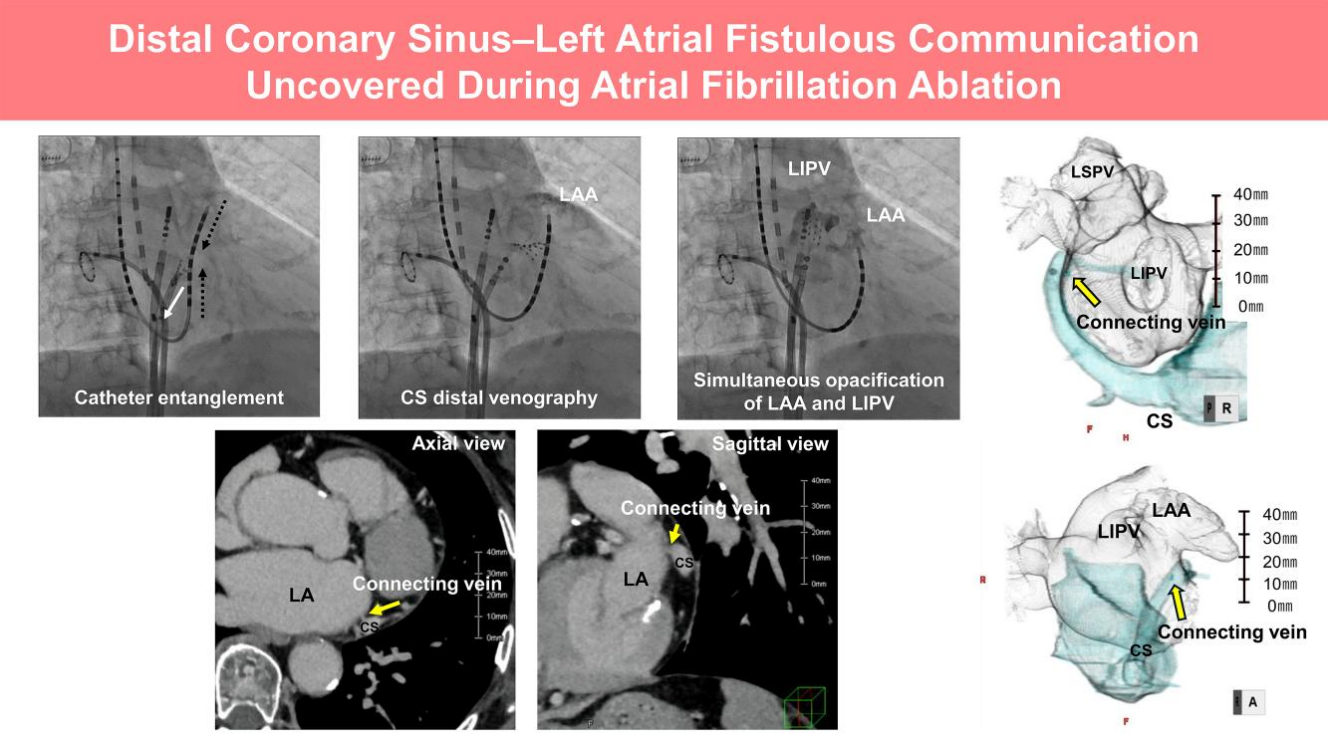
Distal CS–left atrial fistulous communication identified during atrial fibrillation ablation. Coronary sinus distal venography showed opacification of the LAA with simultaneous LIPV filling, and CT (axial and sagittal views) confirmed the connecting vein.
